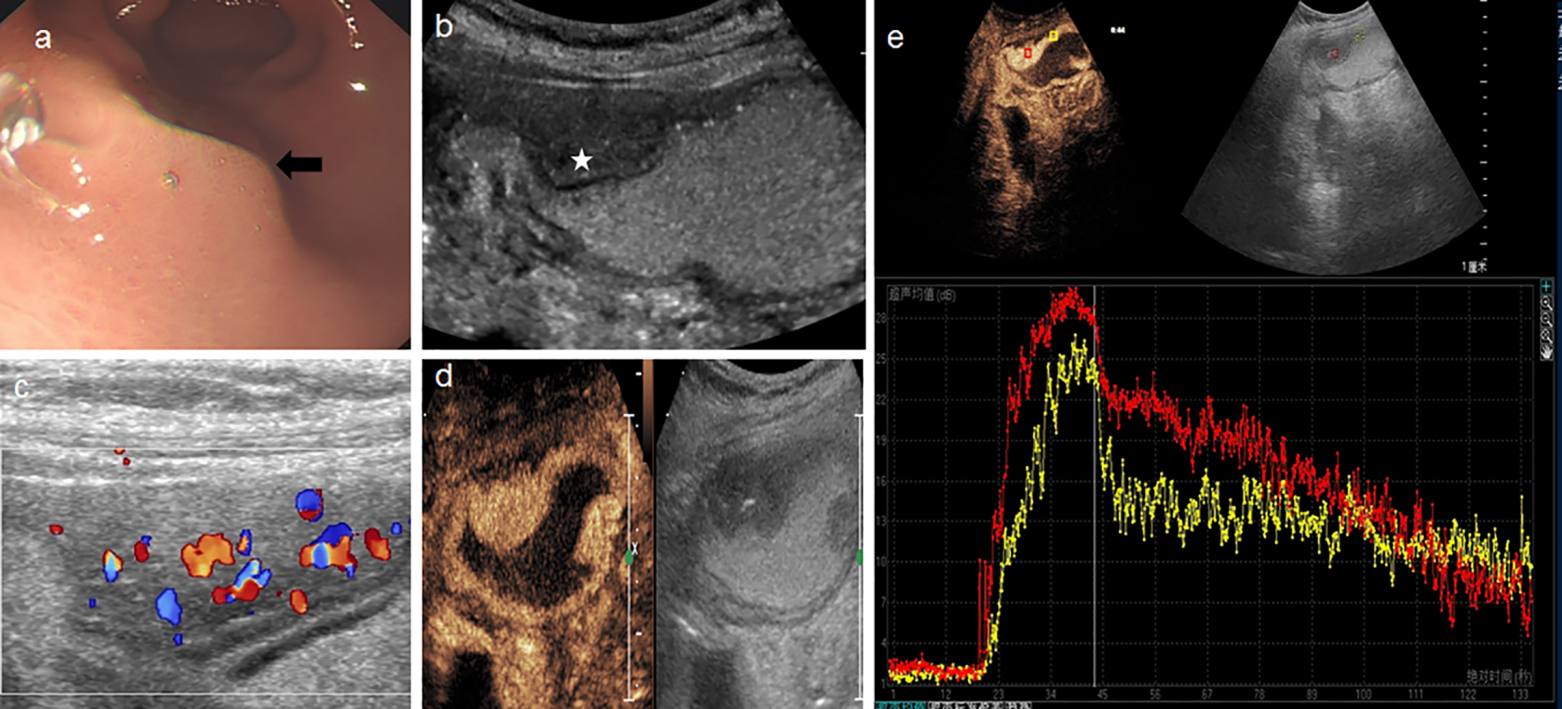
A 59-year-old woman with an inflammatory mass. a: Gastro-endoscope detected a bump with intact overlying mucosa protruding into the cavity. b: Conventional trans-abdominal ultrasound with oral contrast agent showed a well-defined hypoechoic lesion (star), with an intact mucosa. c: multiple vessels were visible on the color Doppler. d: DCUS showed a homogeneous hyper-enhanced mass in the antrum. e: Time-intensity curve depicted the lesion (red line) have higher PI than surrounding normal tissue (yellow).
